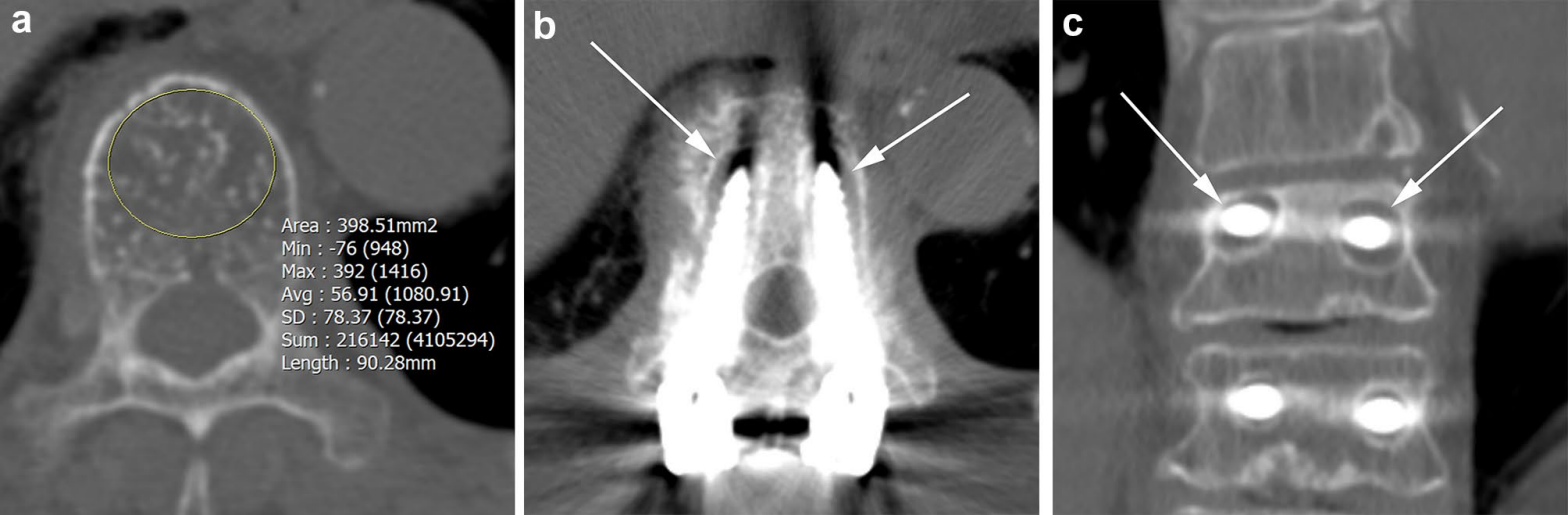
A 76-year-old woman who underwent spinal fusion surgery from T10 to S1. In the axial plane of the preoperative CT scan (a), the Hounsfield unit value of the upper instrumented vertebral body (T10) was 56.91, which was lower than the cutoff value. Postoperative CT images performed 1 year after surgery revealed bilateral pedicle screw loosening in both the axial (b) and coronal (c) planes (arrows). *min* minimum, *max* maximum, *avg* average, *SD* standard deviation.
